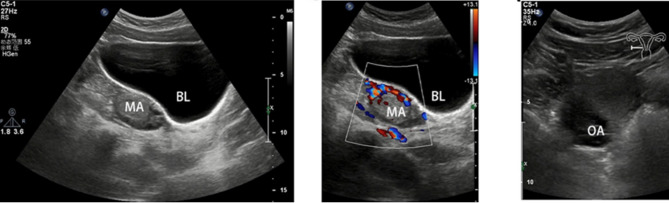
Ultrasound images. Mass (MA) on the left ovary (left and middle panels). Right ovary (OA) displayed characteristics of polycystic ovary syndrome (right panel). BL: Bladder
